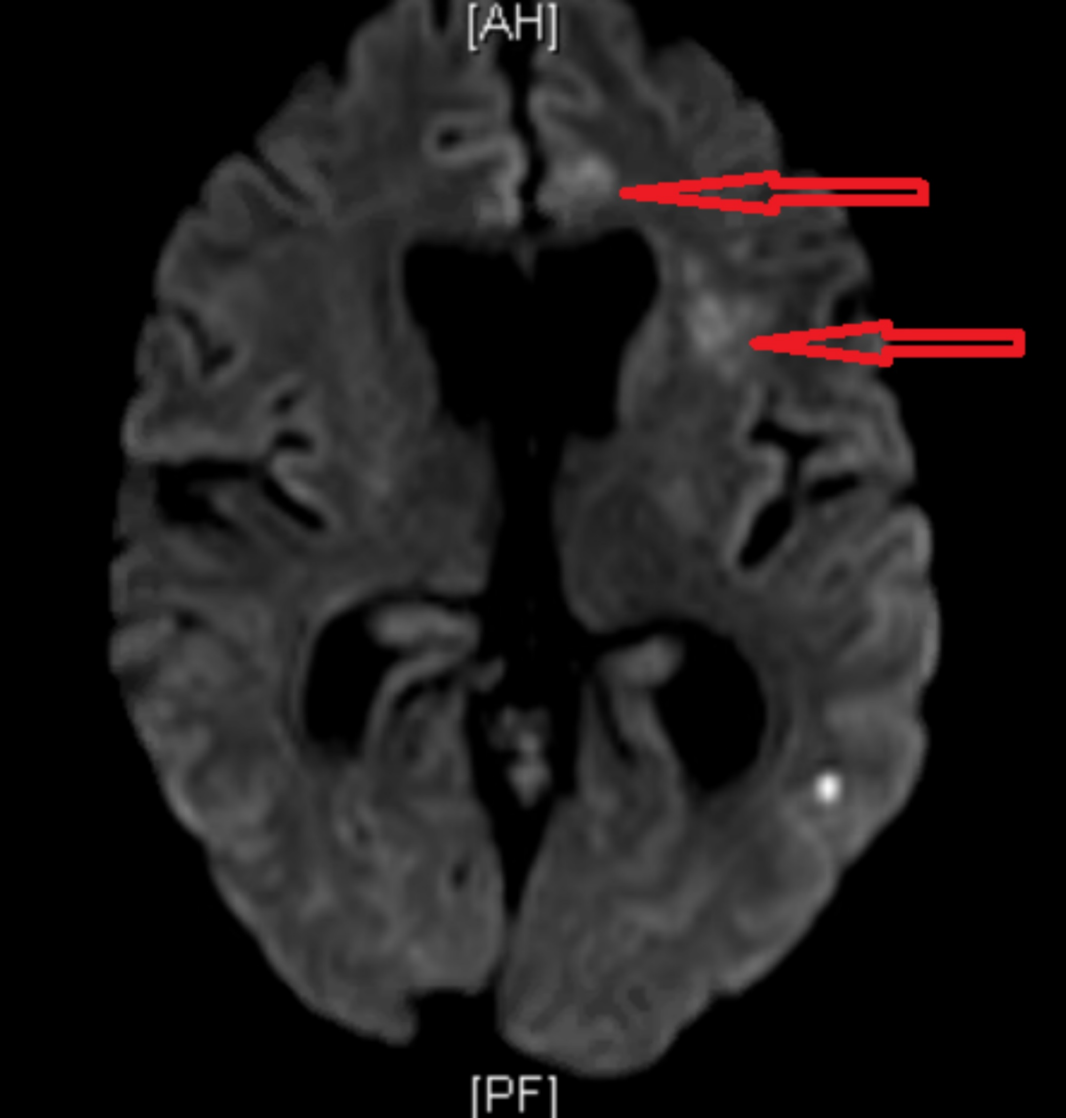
Magnetic resonance imaging (MRI) of the brain showing a left frontal and temporal lobe infarct
